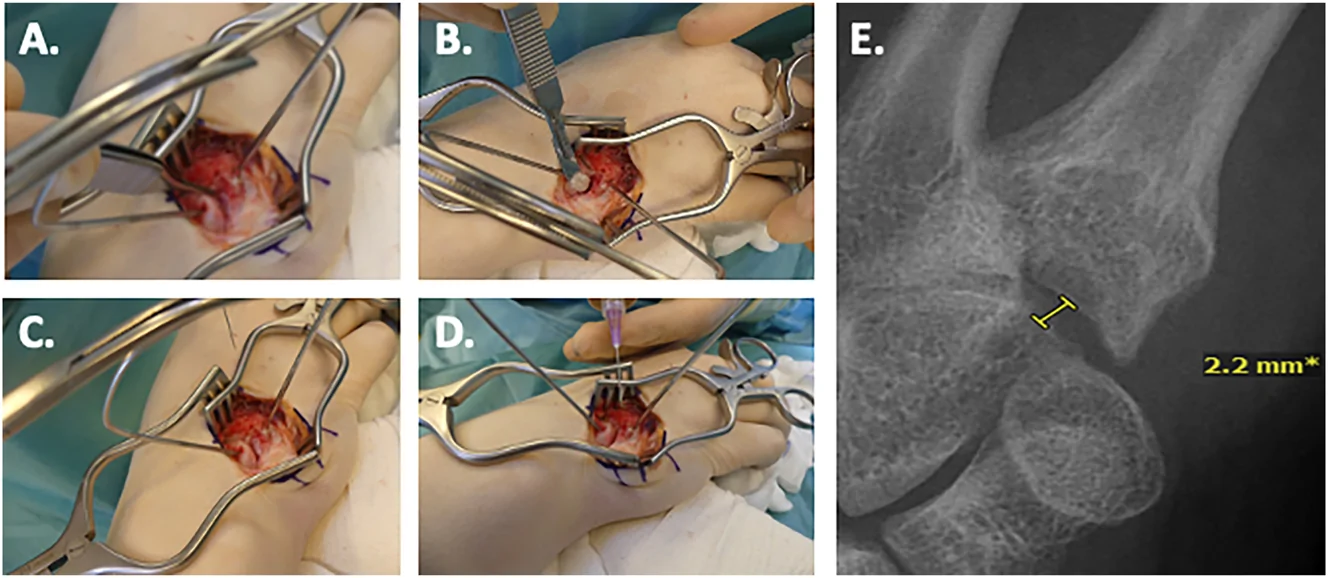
CMC arthroplasty with minced cartilage. (A) Intraoperative view after joint debridement, with forceps indicating the CMC-V joint. (B) Cartilage fragments mixed with PRP prior to transplantation into the cartilage lesion. (C) Transplanted minced cartilage at the base of metacarpal V. (D) Application of thrombin solution to secure the cartilage graft. (E) Postoperative X-ray image of the CMC joint (joint space measured at 2.2 mm).
